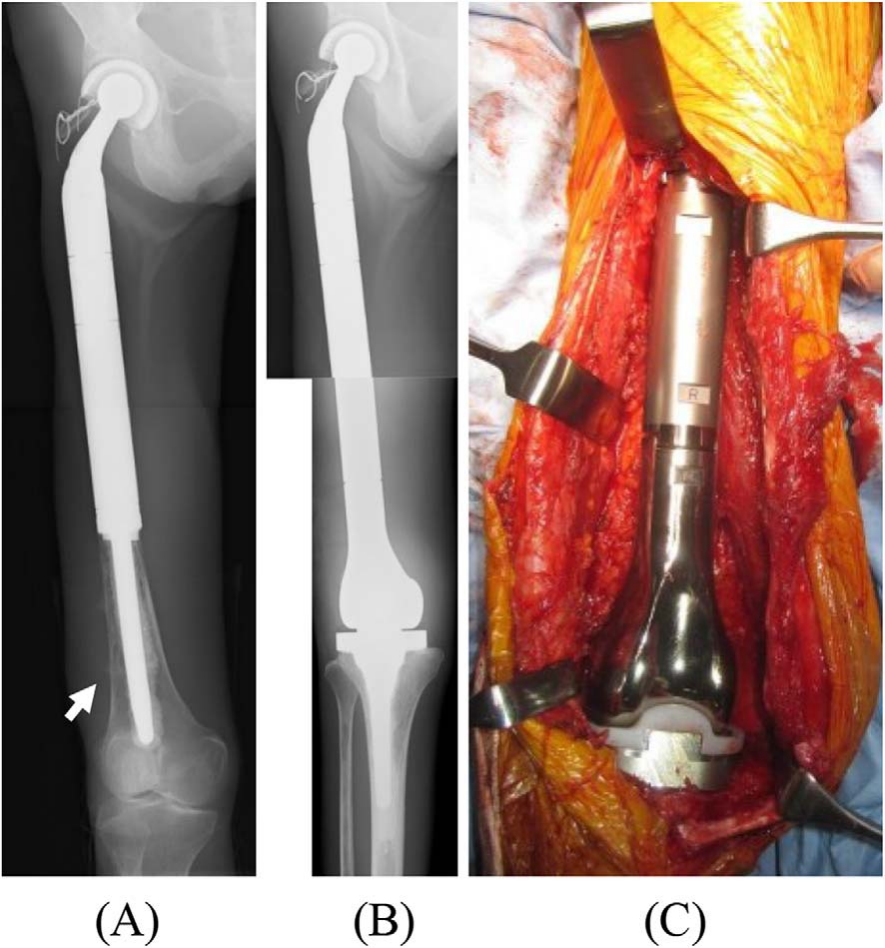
(A) X-ray of the right femur of 46-year-old woman with a local recurrence (arrow) of metastatic bone tumor from breast cancer after PFR. (B) Radiograph demonstrating the reconstruction of the right femur after TFR. (C) An intraoperative photograph after reconstruction of femoral bone using a TFR.
